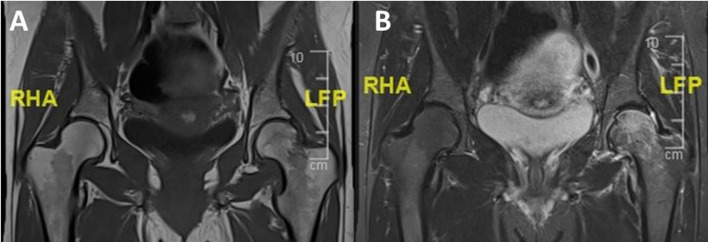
MRI performed at 27 weeks of gestation showed a decreased signal intensity in the proximal femur on T1-weighted imaging (A) and an increased signal on the T2-weighted imaging sequence. (B) MRI; Magnetic Resonance Imaging.
